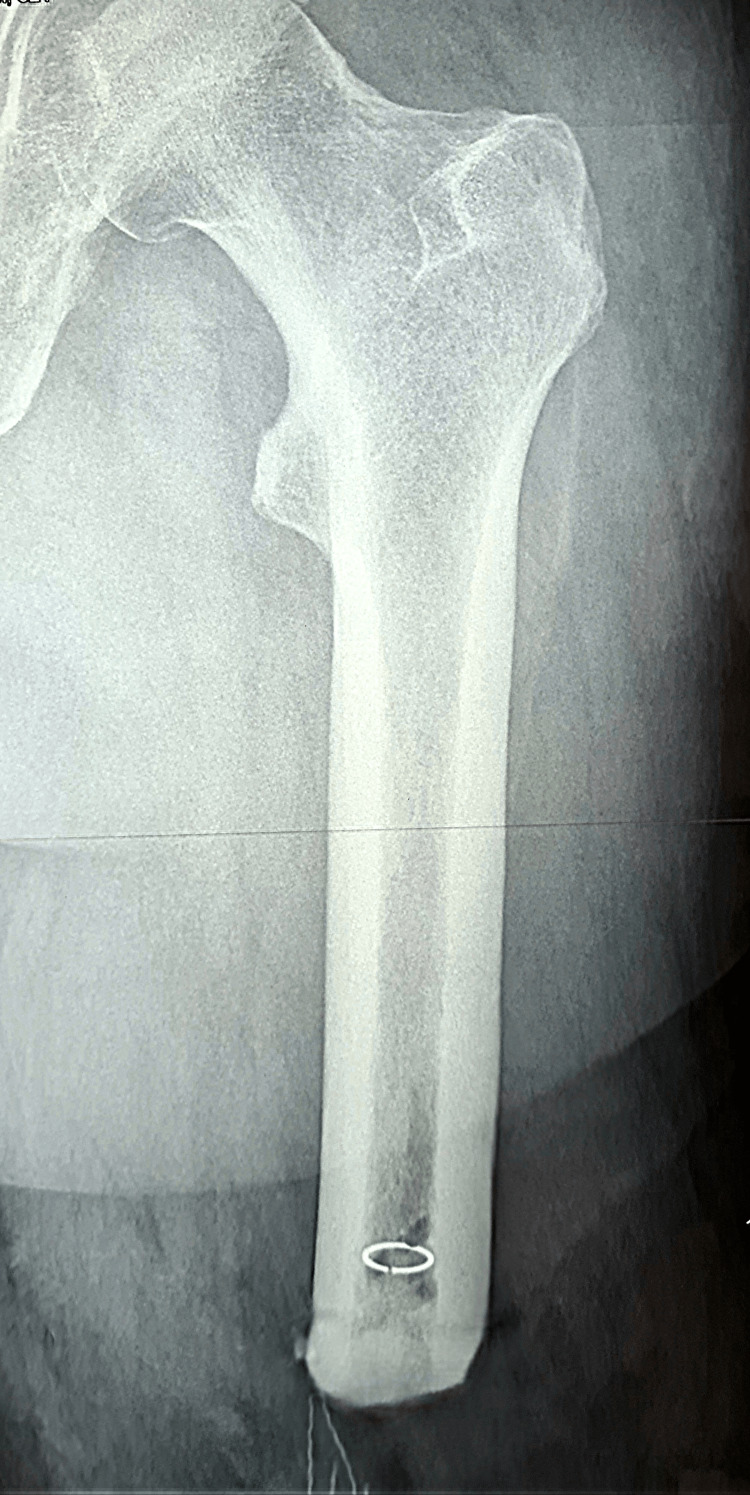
Antero-posterior (AP) radiograph of the AKA
